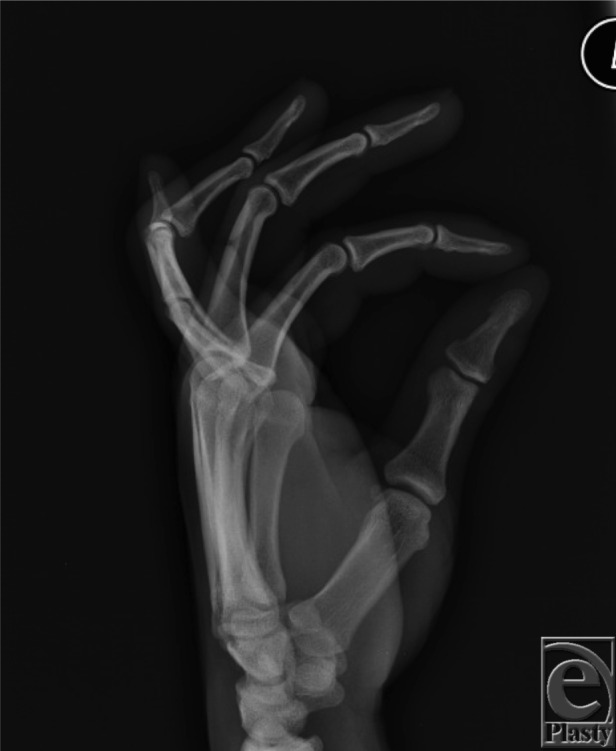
Radiograph demonstrating a *boutonnière* deformity (late) with the proximal interphalangeal joint in flexion and the distal interphalangeal joint in hyperextension.
